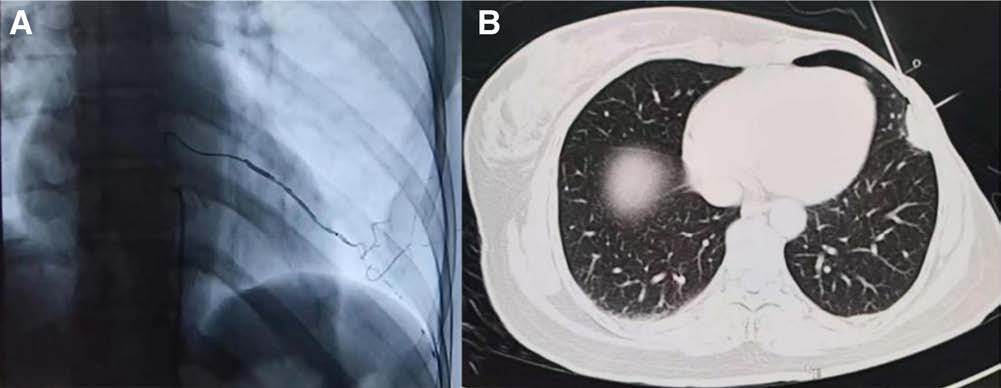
Intraoperative imaging of combined transarterial embolization and radiofrequency ablation. (A) Fluoroscopic image demonstrating selective catheterization of the tumor-feeding intercostal artery and embolization with lipiodol and microspheres during transarterial embolization. (B) Axial CT image obtained during percutaneous radiofrequency ablation, showing electrode placement within the lesion and the surrounding hydro-dissection fluid to protect adjacent pleura and intercostal nerves.
